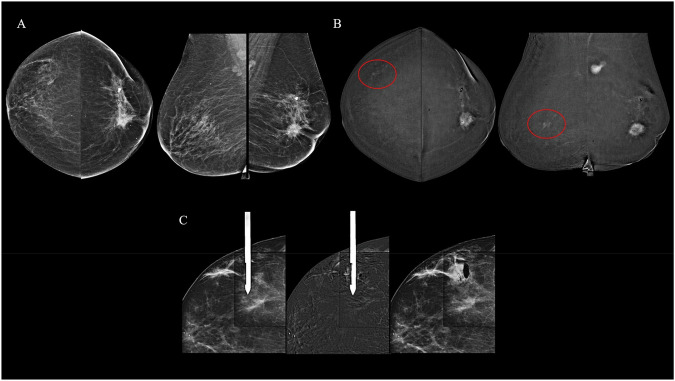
Preoperative CEM for locoregional staging in a 58-year-old woman with node-positive invasive ductal carcinoma of the left central outer quadrant. LE images (A) show the known index lesion in the left breast. Recombined images (B) demonstrate enhancement of the index lesion in the left central outer quadrant and an additional suspicious non-mass enhancement in the right upper outer quadrant (red circle). The area showed no correlation at second-look ultrasound or digital breast tomosynthesis. C CEM-guided biopsy of the enhancing area was therefore performed, yielding a diagnosis of DCIS
